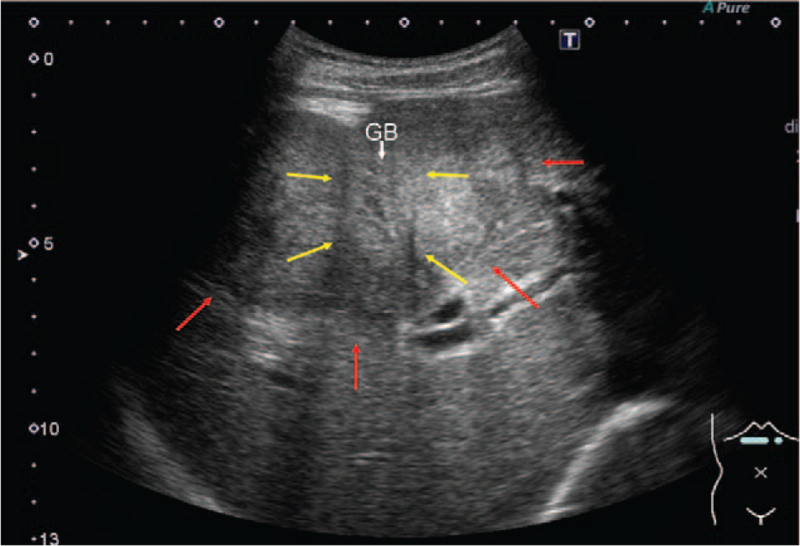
Abdominal ultrasonography findings at the time of diagnosis. Yellow arrow: the gallbladder with its narrowed lumen. Red arrow: a hyperechoic mass with an unclear border in the liver suspected of being connected to the gallbladder. GB = gallbladder.
